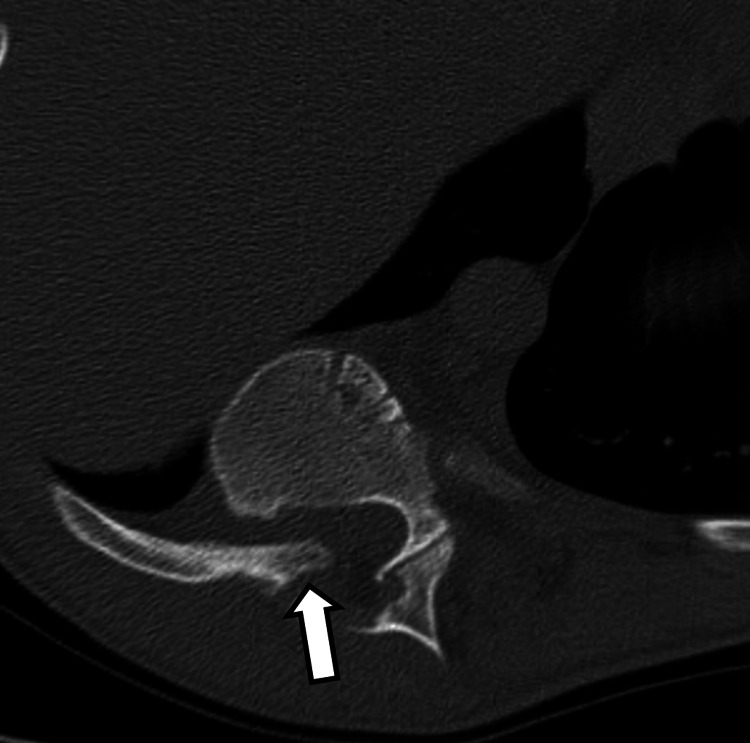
Axial CT images in bone kernel through T10-T11 neural foramen showing protrusion of the pointed head of the rib through the foramen into the spinal canal (arrow).
